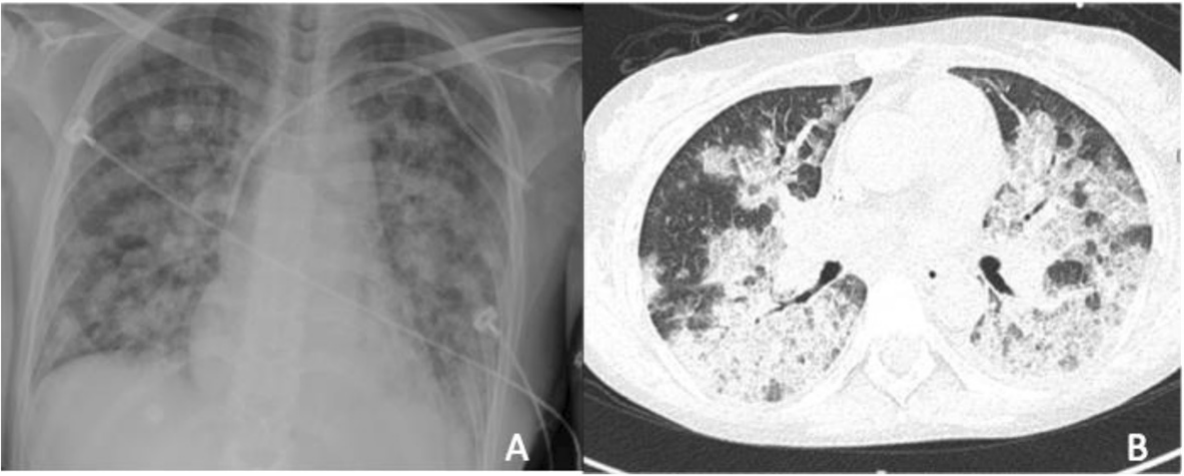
Chest X-ray and CT scan. (A) Chest X-ray where bilateral pulmonary infiltrates were observed. (B) Computed axial tomography (CT) scan where mixed basal pulmonary infiltrates and alveolar collapse were observed. Images were taken at the time of admission (day 1).
